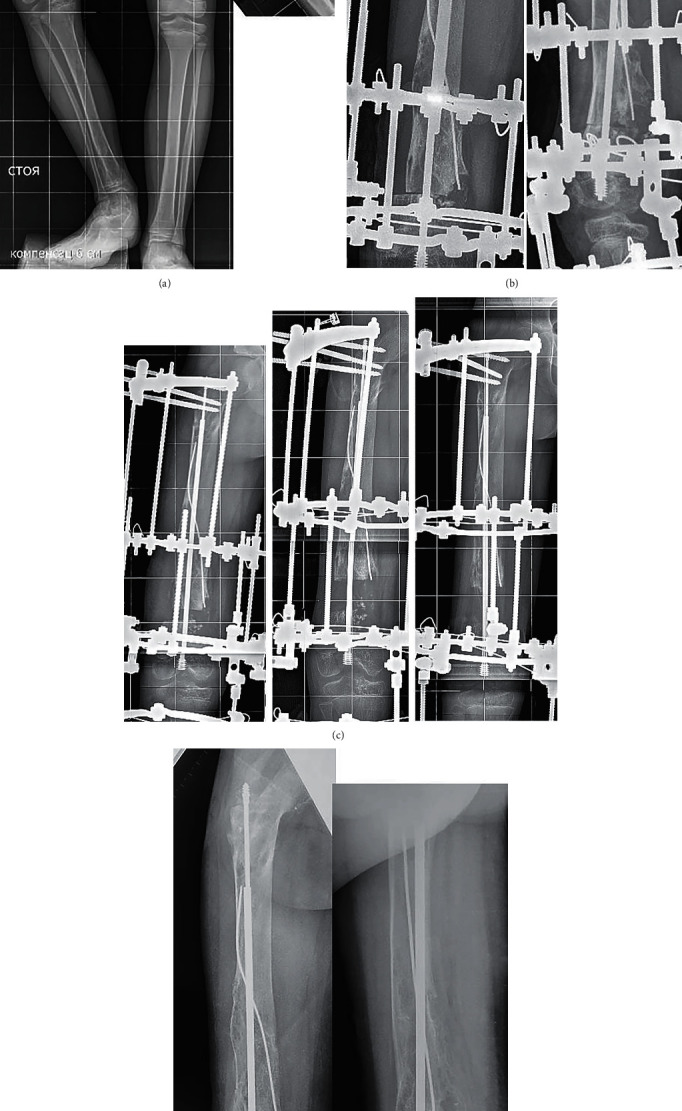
Retrograde telescoping rod for femoral lengthening in patent R.: (a) preoperative full-size standing radiograph and lateral view of the right femur. Note a voluminous distal cartilaginous zone; (b) intraoperative radiograph demonstrating retrograde position of rod and exact centration of knee joint center and mechanical femoral shaft axis; (c) consecutive radiographs of distraction period; and (d) after frame removal.
